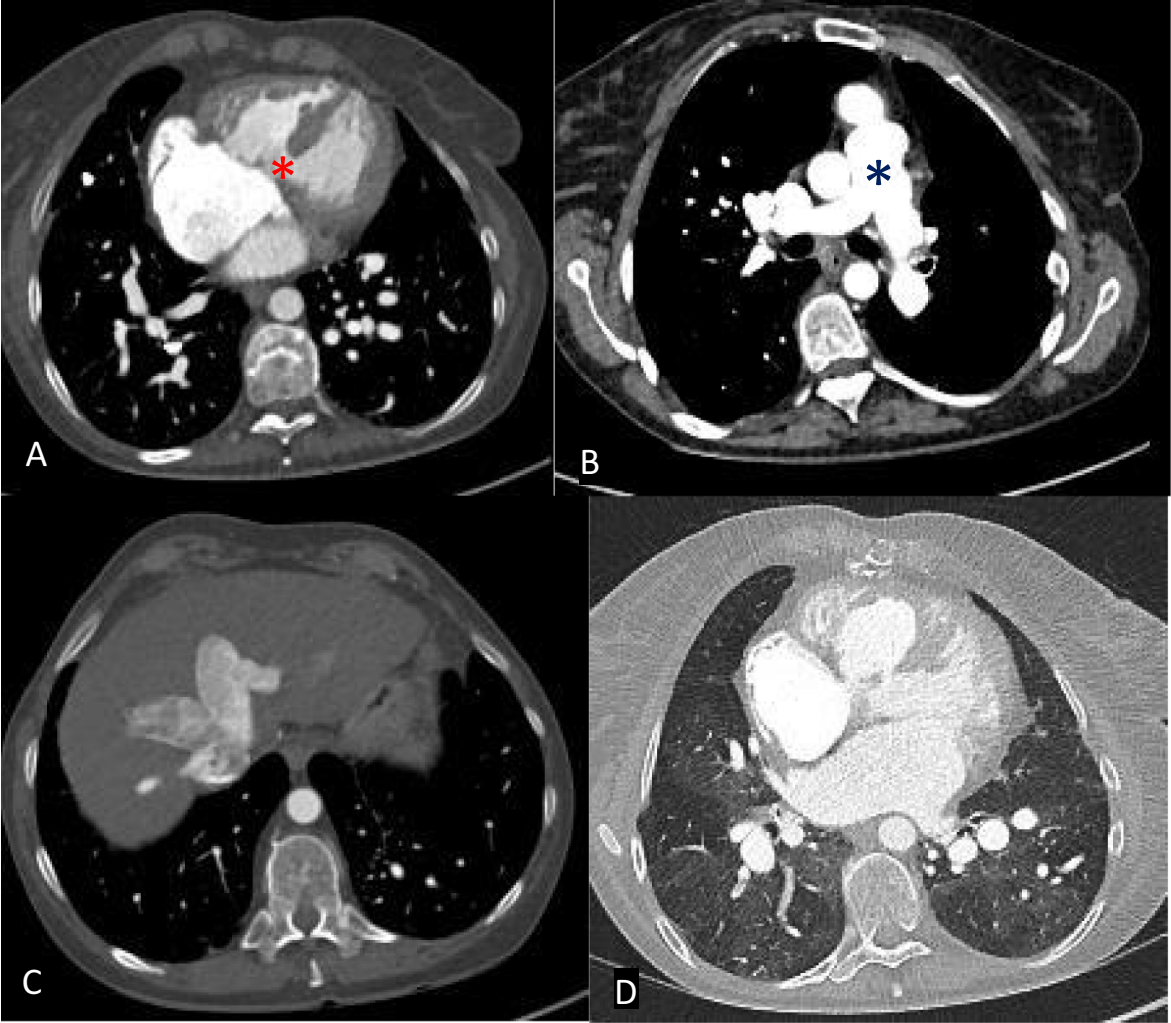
Select axial CT images in the bone (A and C), soft tissue (B), and lung windows (D). A Large perimembranous VSD (red asterisk) and right ventricular hypertrophy. B Dilated pulmonary trunk (blue asterisk) C reflux of contrast into the IVC and hepatic veins D bilateral pulmonary parenchymal mosaicism. Findings are compatible with pulmonary hypertension and right ventricular heart strain due to a long-standing VSD. Although this is an example of an isolated VSD, similar features of pulmonary hypertension can be seen in large residual VSDs in the setting of repaired TOF or repaired D-TGA with a VSD
